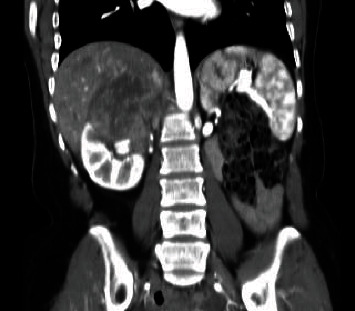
Coronal CT view of the lesion, showing the involvement of the right kidney and IVC.
